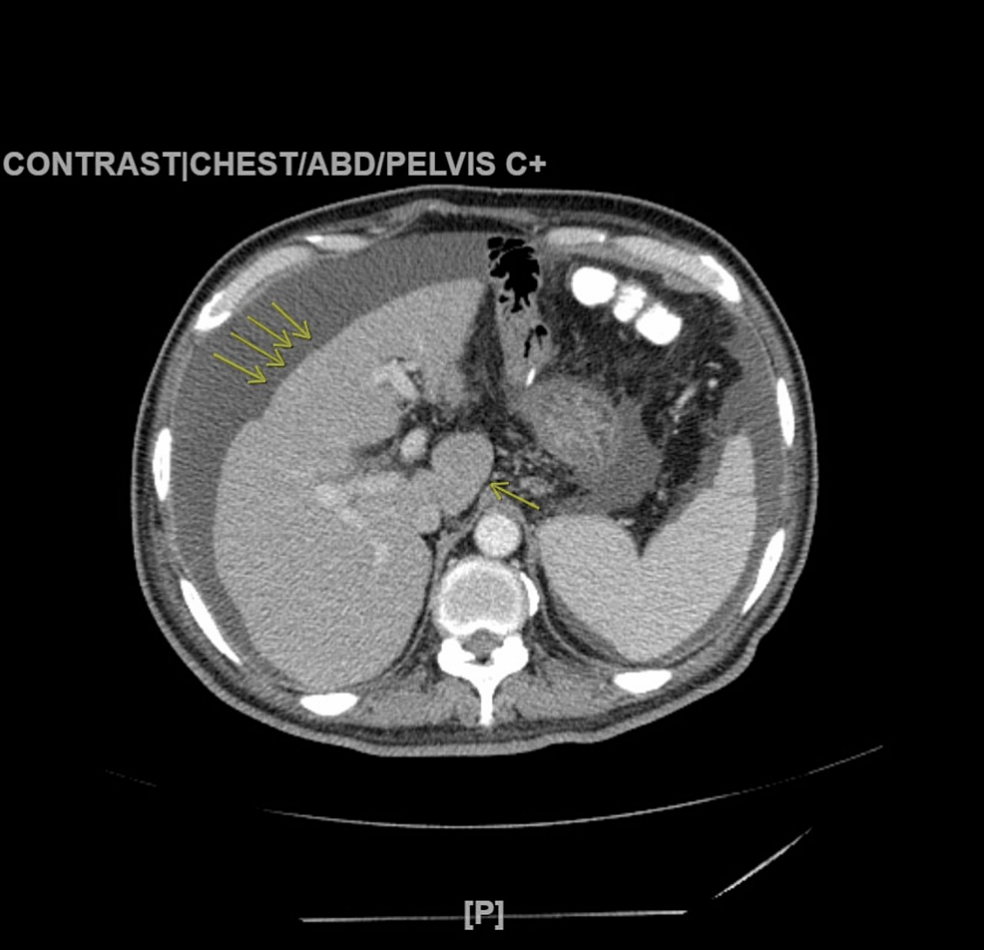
CT abdomen with multiple arrows showing the nodular shrunken and cirrhotic liver; the single arrow on the right pointing to the enlarged caudate lobe.
